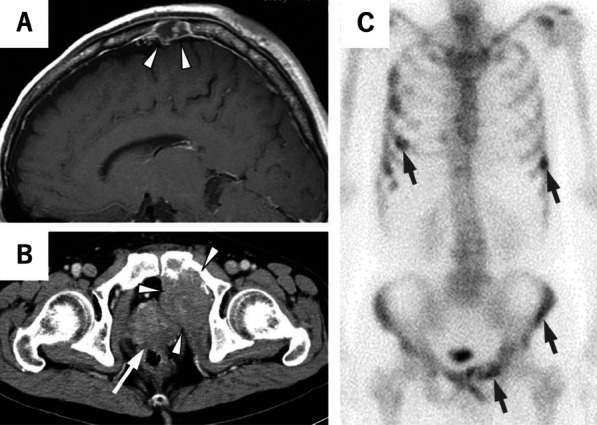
Imaging at diagnosis. A Magnetic resonance image of a tumor in the patient’s skull. B Computed tomography scan image showing osteolytic lesions on the pubis, adjacent to the abnormally enlarged prostate. C
